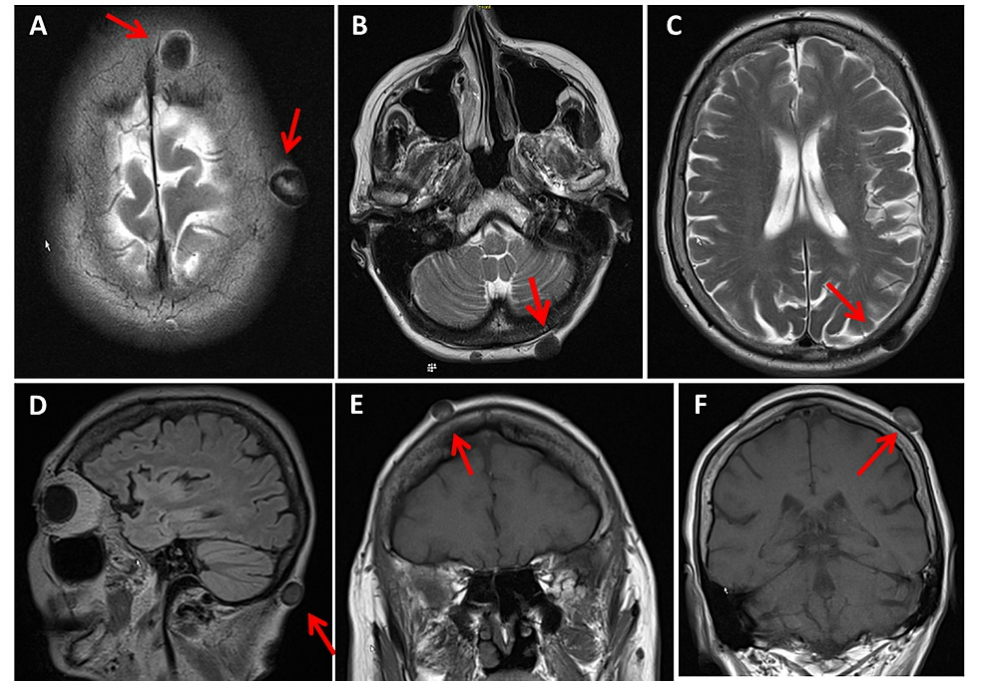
MRI of the brain showing multiple atheromas irregularly distributed over the scalp A to C: axial images showing atheromas at supra- and infratentorial levels, D: sagittal image showing a nuchal atheroma, E and F: coronary views showing atheromas lateral to the vertex
